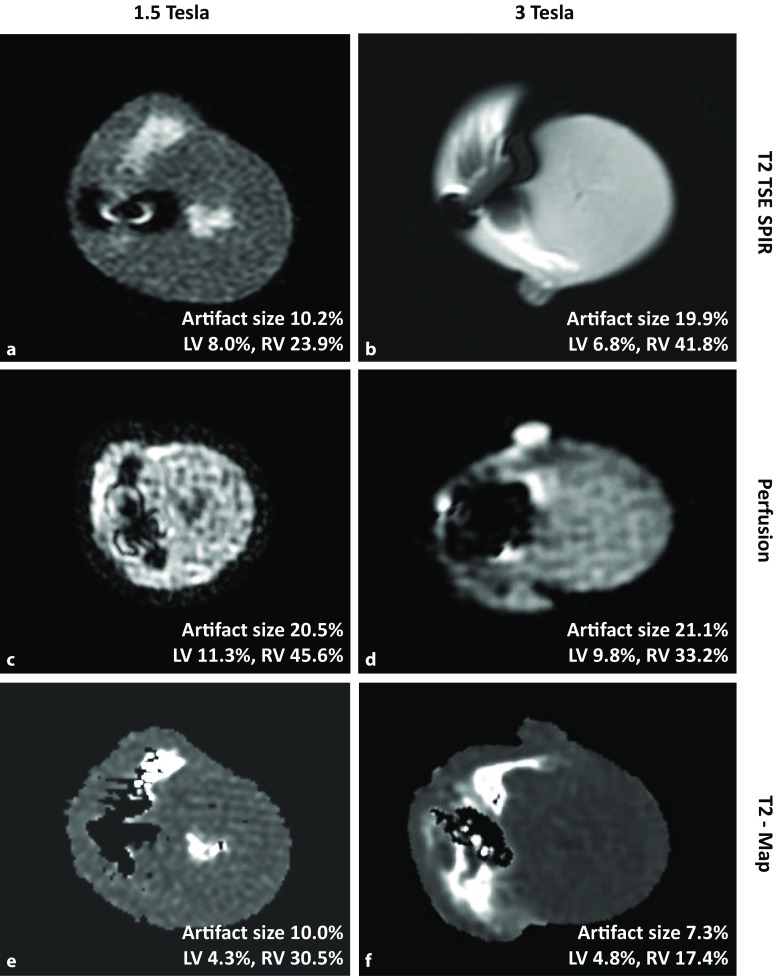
Magnetic resonance imaging T2 TSE-SPIR (a, b), perfusion (c, d) and T2 (e, f) sequences showing few severe artifacts in the perifocal area
